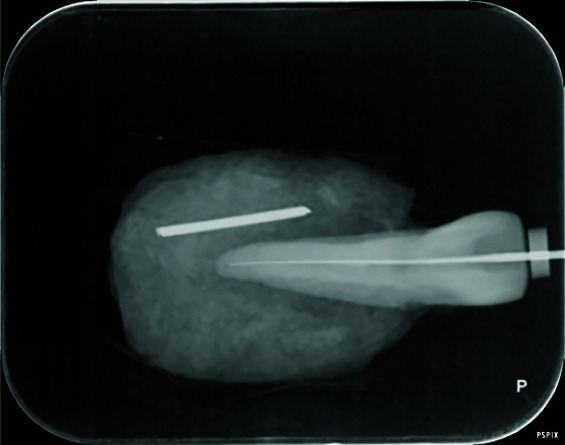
Digital radiograph with sharpness filter
